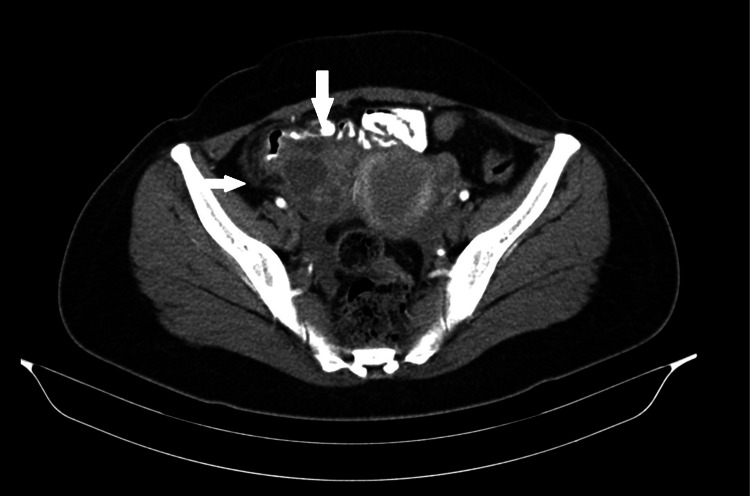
CT scan of the patient revealed a complex cystic mass in the anatomic region of the right ovary
